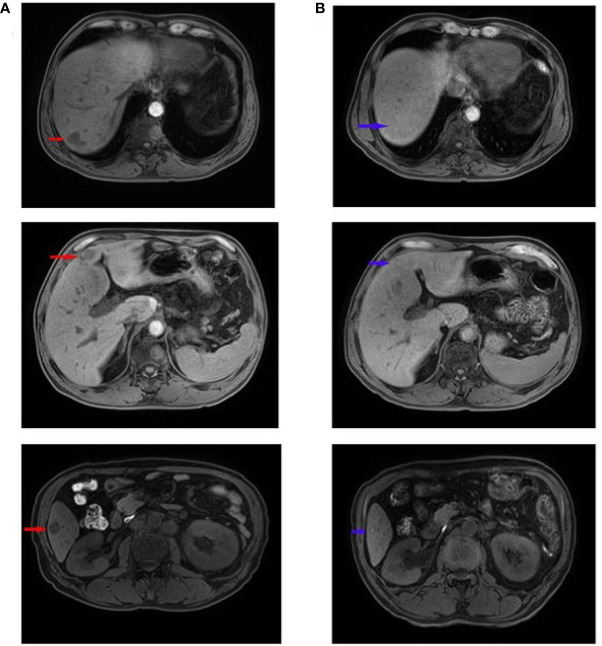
MRI images before and after combinative treatment. (A) Liver MRI metastases before the treatment of apatinib combined with camrelizumab and TAS-102. The larger one (with a size of about 2.5×1.7 cm) was located in the left medial lobe. The red arrow indicates the primary liver metastases. (B) Liver MRI metastases after the comprehensive treatment of apatinib combined with camrelizumab and TAS-102. The larger one (a diameter of about 1.0 cm) was located in the posterior upper segment of the right lobe of liver. The blue arrow indicates that the liver metastases shrunk or disappeared.
